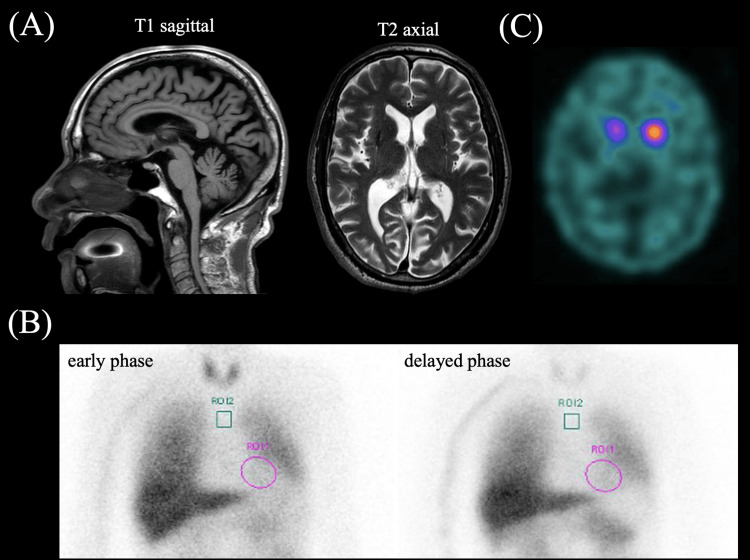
Neuroimaging findings in the patient (A) Brain magnetic resonance imaging and angiography revealed no structural abnormalities; (B) Cardiac ^123^I-metaiodobenzylguanidine scintigraphy demonstrated a reduced heart-to-mediastinum (H/M) ratio at the initial evaluation (early H/M ratio, 1.985; delayed H/M ratio, 1.647; washout rate, 50.5%). The institutional reference values were early and delayed H/M ratios >2.0 and a washout rate <34%; (C) Dopamine transporter imaging revealed bilaterally reduced specific binding ratios (right, 2.48; left, 3.10).
